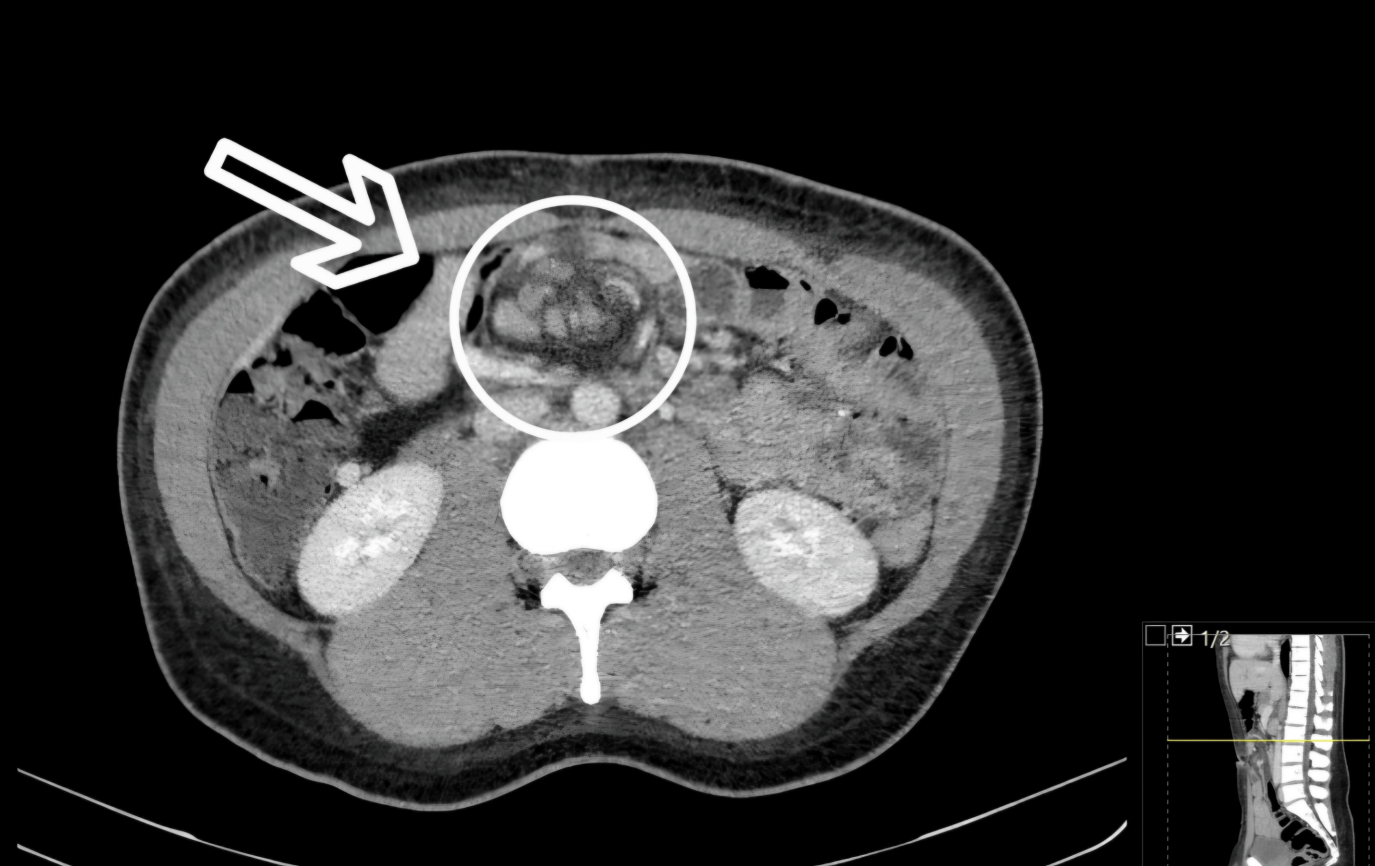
Case 1 - Axial CT scan showing swirl sign suggestive of internal hernia. Contrast-enhanced axial CT image demonstrates the classic “swirl sign” of mesenteric vessels (white circle), consistent with internal herniation. The white arrow highlights the twisted mesenteric fat and vessels, a key radiologic feature in diagnosing Petersen’s hernia.
